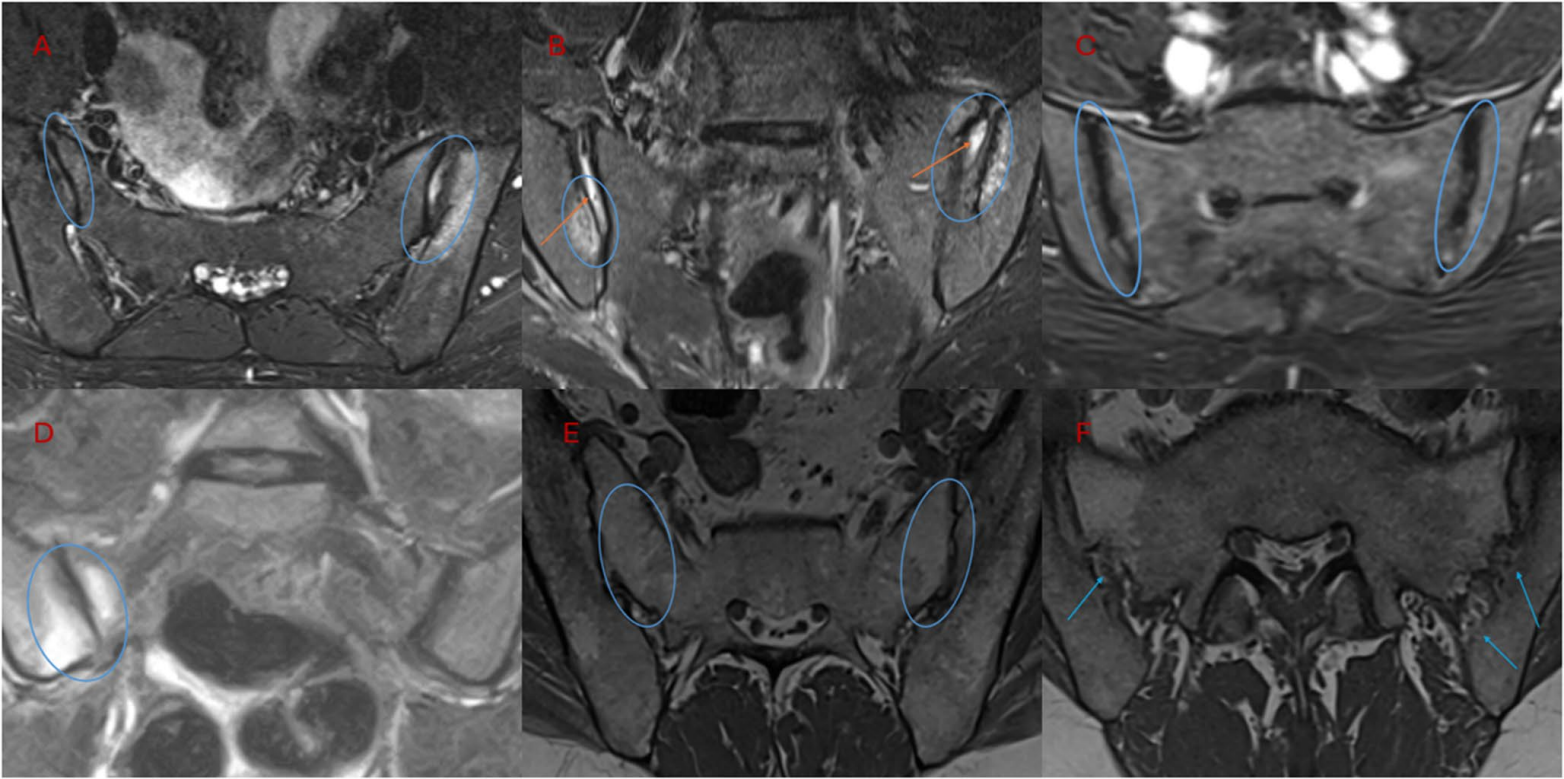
A Axial STIR sequence: sacroiliitis in axial spondyloarthritis showing bilateral high signal intensity consistent with bone marrow edema, more pronounced on the left side. B Axial STIR sequence: postpartum bone marrow edema at the sacroiliac joints (loop), with joint distraction (arrows) and fluid in the joint space. C Axial STIR sequence: osteitis condensans ilii with bilateral iliac sclerosis and mild sacroiliac joint irregularity, without associated bone marrow edema. D Axial STIR sequence: sacroiliac joints in a young female with inflammatory back pain. MRI confirms classical right-sided active sacroiliitis with subchondral bone marrow edema, consistent with early axial spondyloarthritis. In advanced disease, changes typically become bilateral and symmetrical. E Axial T1 sequence: fat metaplasia presenting as homogeneously bright signal in the subchondral bone. F Axial T1 sequence: classical bilateral iliac erosions (arrows), characterized by loss of cortical bone definition
